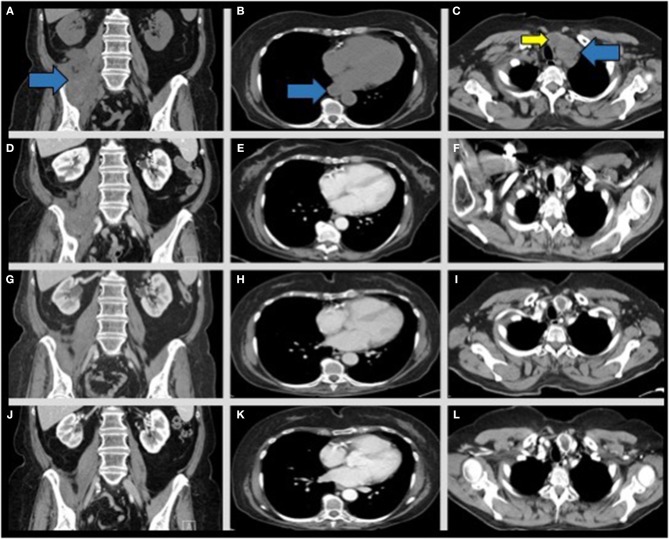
CT chest/abdomen/pelvis scans from post-radiation disease progression through continued interval follow up to monitor late responses to radiation therapy and to assess for recurrence. Representative coronal images from interval CT scans of the pelvic mass (left column- blue arrow), para-esophageal (middle column- blue arrow), and left biopsy-proven supraclavicular lymph node (right column- blue arrow) across several interval CT scans. (A–C) 1 month post-radiation without contrast. (D–F) 5 months post-radiation with contrast, (G–I) 7 months post-radiation with contrast, (J–L) 17 months post-radiation with contrast. Note imaging appearance of a stable, regressed right retroperitoneal mass (D, blue arrow), regression of the enlarged para-esophageal lymph node (E, blue arrow), and regression of the left supraclavicular lymph node noted at 5 months post-radiation (F, blue arrow). A previously identified enlarged left thyroid nodule is also present across the scans (C,F,I,L, yellow arrow) and is not consistent with metastatic disease.
